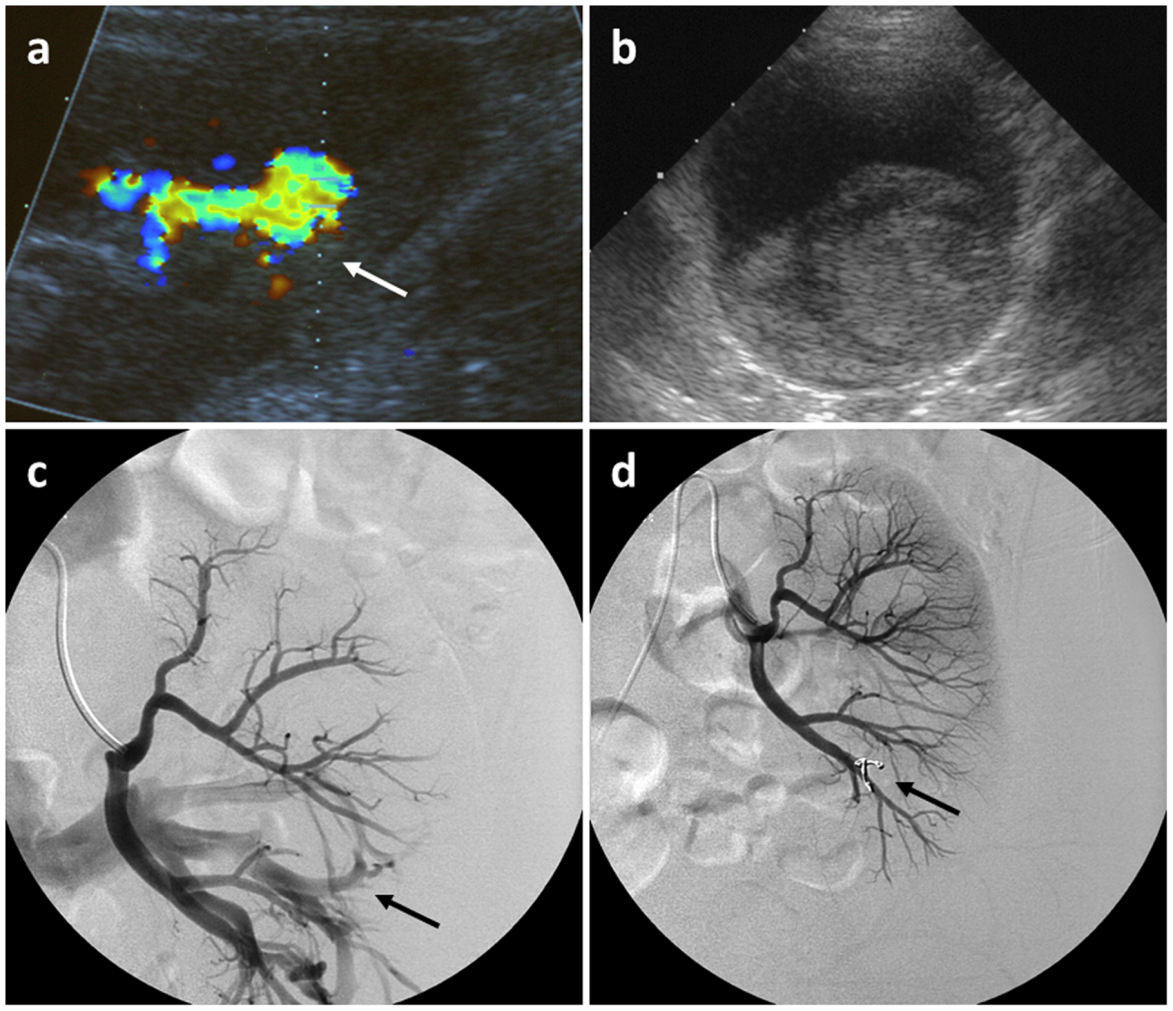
Ultrasound findings of an arteriovenous fistula (a, white arrow) and a bladder tamponade (b). AV-fistula was angiographically embolized (c and d, black arrow) (10 year old patient, male).
